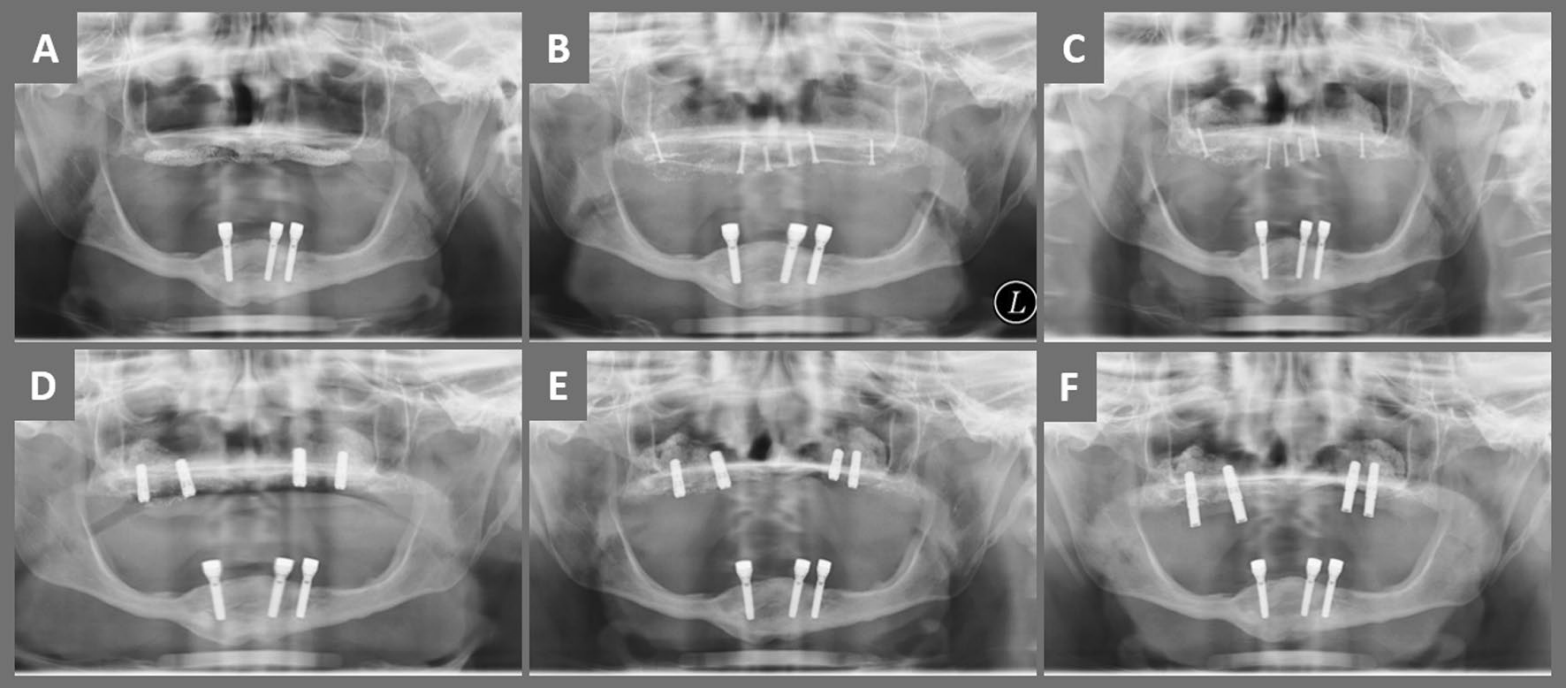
Panoramic radiographs: A preoperative, B immediately after augmentation, C after 3 months, D 5 months postoperatively after removal of the mini screws and implant insertion, E 11 months and F 23 months postoperatively
